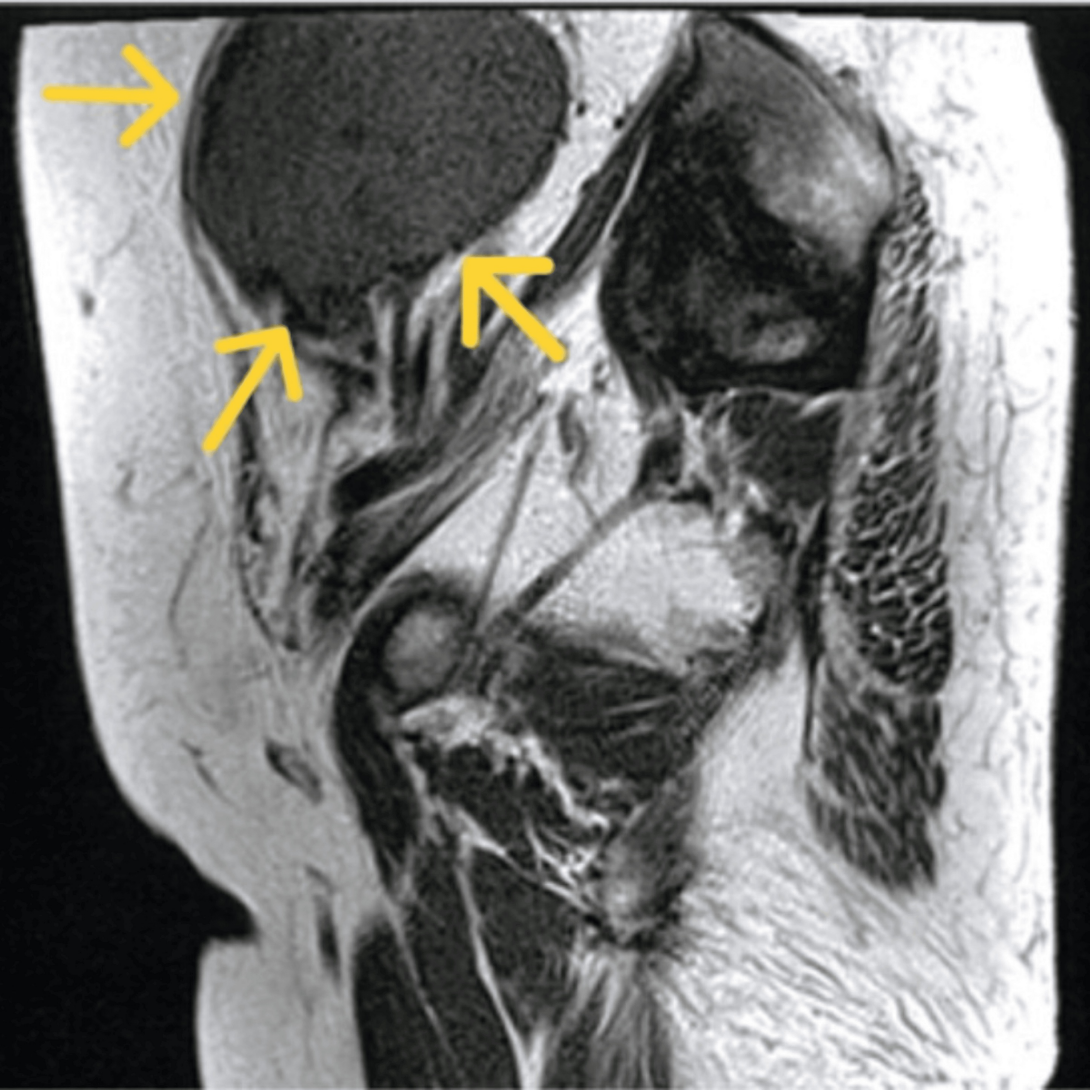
T2-weighted MRI scan. MRI demonstrating a 76 × 56 mm mass (yellow arrow) with slightly higher T2 signal intensity than the myometrium and low T1 signal intensity.
